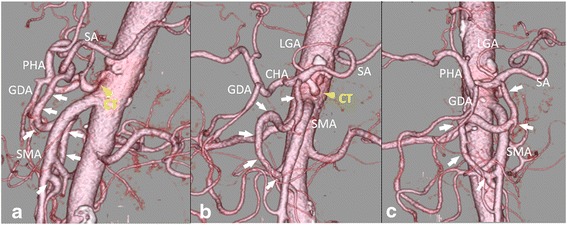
a–c A three-dimensional reconstructed computed tomography image showing the arc of Buhler. The *yellow arrowhead* indicates stenosis of the celiac trunk, and the *arrow* indicates the arc of Buhler. *CHA* common hepatic artery, *CT* celiac trunk, *GDA* gastroduodenal artery, *LGA* left gastric artery, *PHA* proper hepatic artery, *SA* splenic artery, *SMA* superior mesenteric artery
